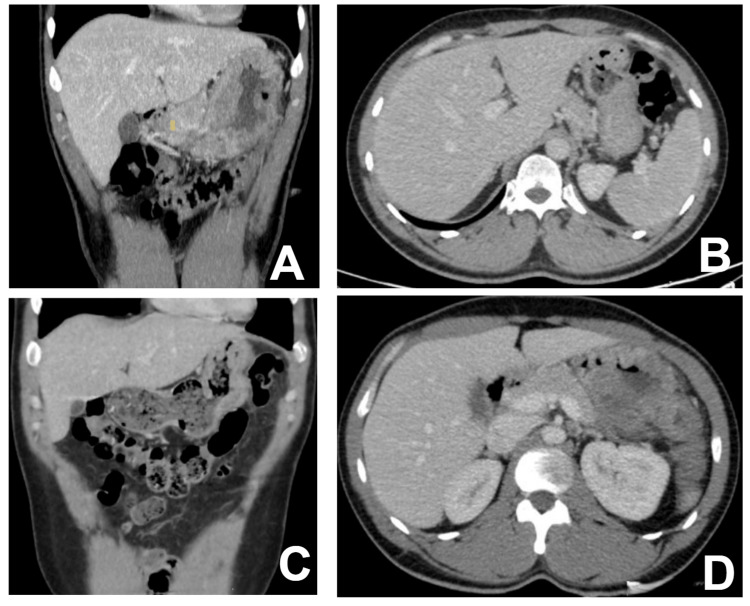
Radiological findings (A,B) First CT examination in March 2022. (C,D) Follow-up CT examination after medical treatment in January 2023. Axial (A) and coronal (B) images were acquired in a portal phase after IV iodinated contrast administration. There is a large gastric tumor presenting as an irregular mural thickening, affecting both the anterior and posterior walls of the gastric body. The inner surface of the mass is irregular due to tumor ulceration, and the posterior gastric wall shows signs of retroperitoneal infiltration with no fat plane with the pancreatic tail. No focal liver lesions or clearly enlarged lymph nodes were detected. Axial (C) and coronal (D) images were acquired in a portal phase after IV contrast injection. The gastric wall is now only minimally thickened in the upper part of the gastric body. The inner surface of the gastric wall is smooth with no clear ulcers, and there is a fat plane between the gastric wall and the pancreas with no radiological signs of retroperitoneal infiltration.
